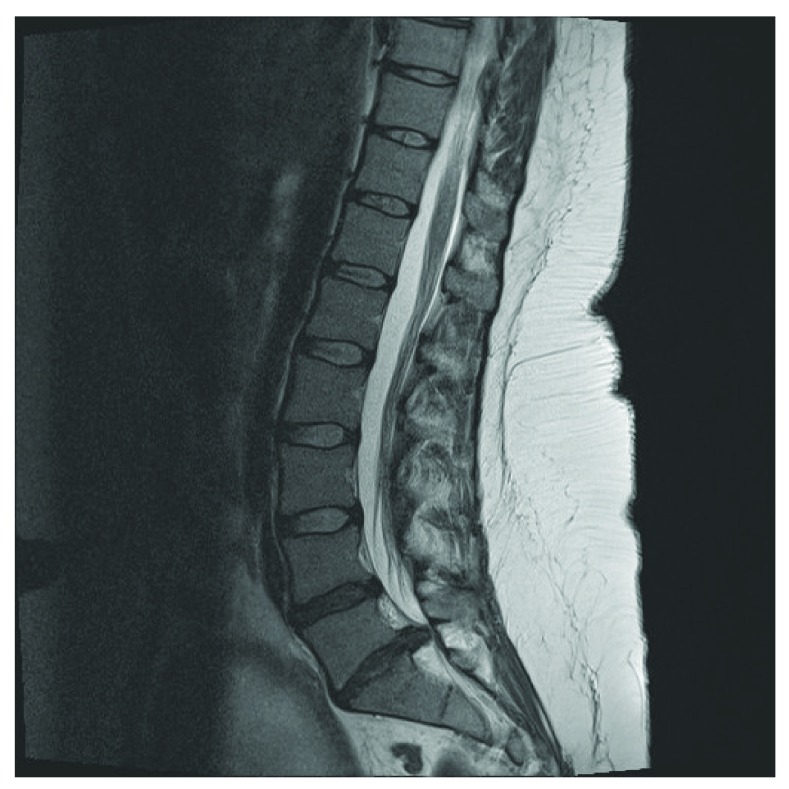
Pre-operative MRI lumbar spine showing a L5/S1 prolapse.
